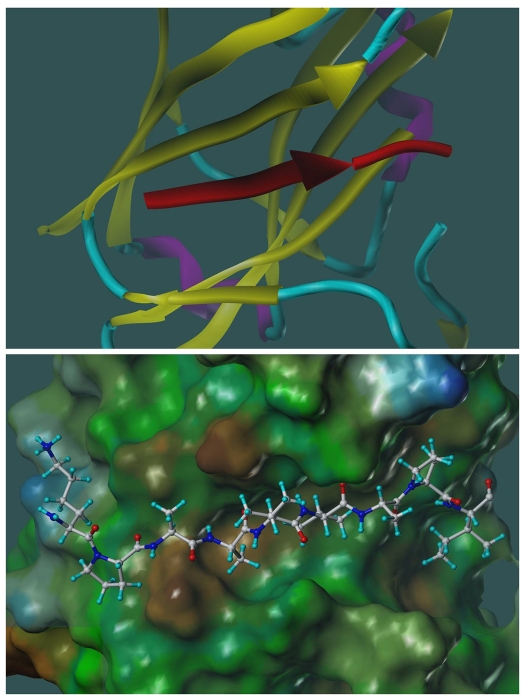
Molecular models representing the X-ray structure of SIAH1 in complex with a SIP derived peptide of sequence EKPAAVVAPITTG (PDB-ID 2A25;) A similar docked geometry was observed in another –X-ray study with SIAH1 in complex with a 24 amino acids Phyllopod peptide (PDB-ID 2AN6). Top panel: ribbon representation of SIAH1 yellow, β-strands; cyan, loop regions, magenta, α-helical regions; the SIP peptide completes a β-strand and it is depicted in red. Bottom panel; SIAH-SIP complex is displayed in the same orientation as in the top panel, but SIAH1 is shown as a surface representation, color coded according to a molecular lipophilicity potential (brown more lipophilic; green, neutral; blue, less lipophilic). The peptide is shown as a ball-and-stick representation. The figure was prepared with MOLCAD as implemented in Sybyl (Tripos, St.Louis).
